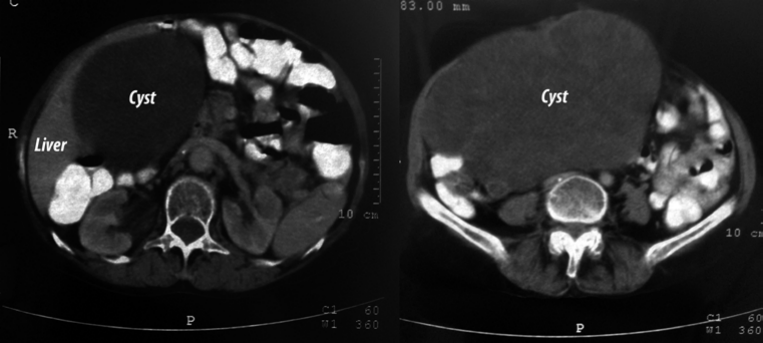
Computed tomography image of the giant cyst.
